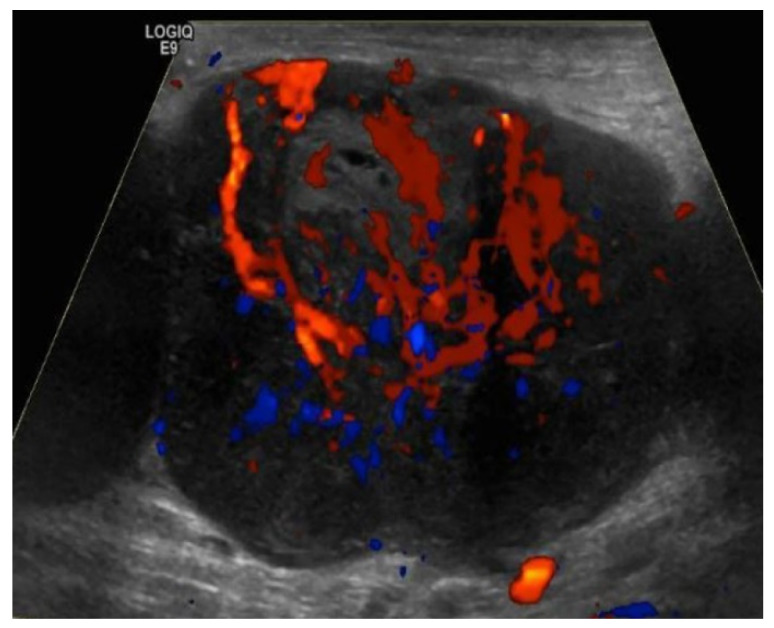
Doppler ultrasound of a right inguinal metastatic lymph node in a 67-year-old male with history of right ankle melanoma shows an enlarged hypoechoic lymph node with mixed (hilar and peripheral) vascularity.
